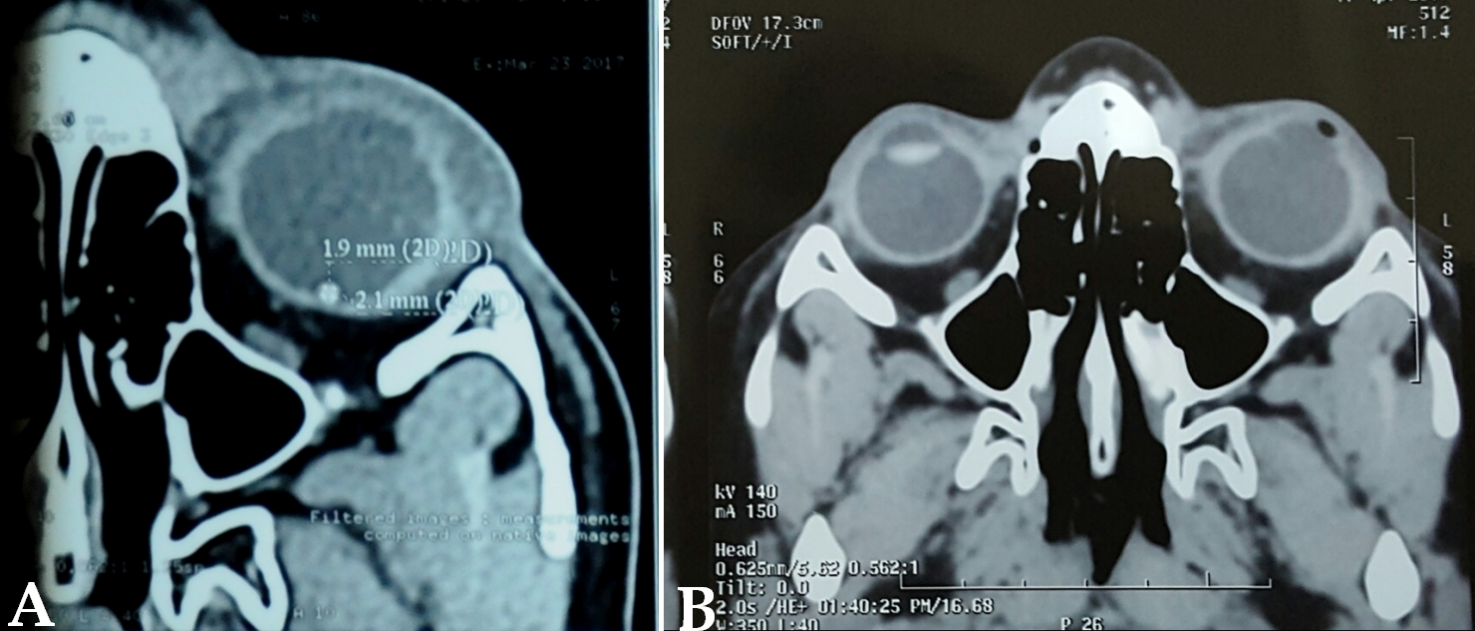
A: CT scan image after the initial surgery showing a round, 1.9 mm x 2.1 mm sized radio dense (400 Hounsfield Units) lesion close to the retina in the left globe. B: CT scan following aspiration of the PFCL bubble showing the absence of any radio dense lesions.
